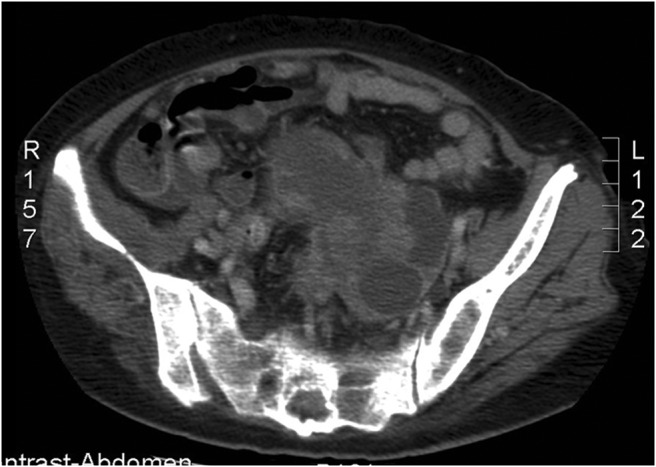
CT scan showing multilobulated cystic lesions over the left adnexa.
